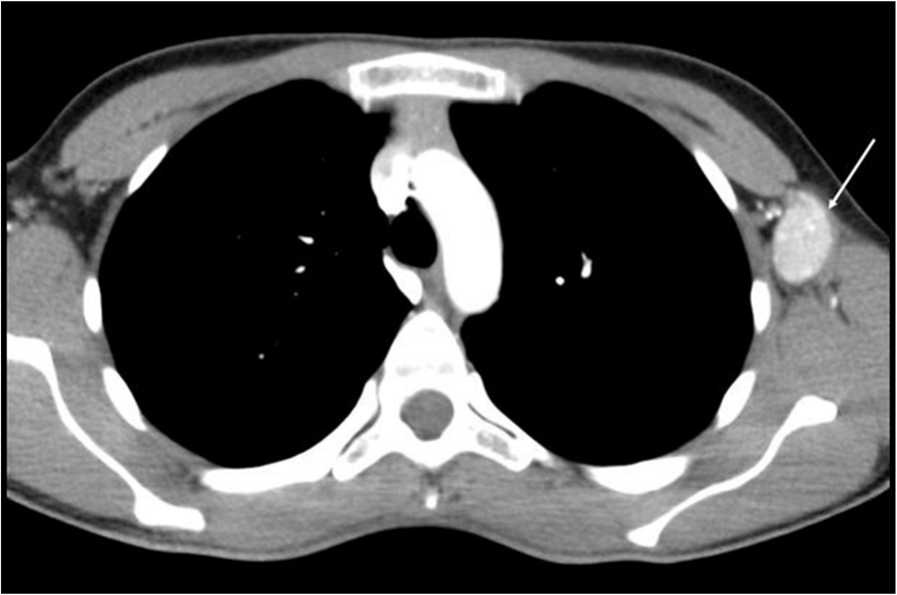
CT scan shows enlarged lymph node (arrow) in the left axilla.
